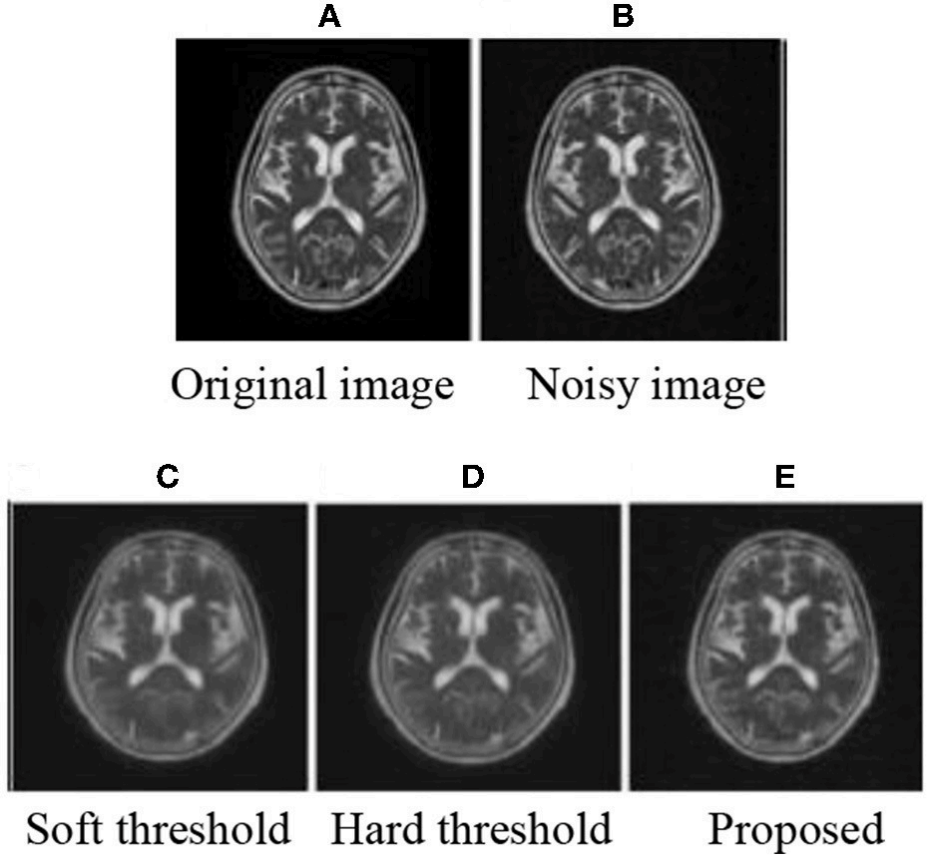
MRI 1 of subjective results.
